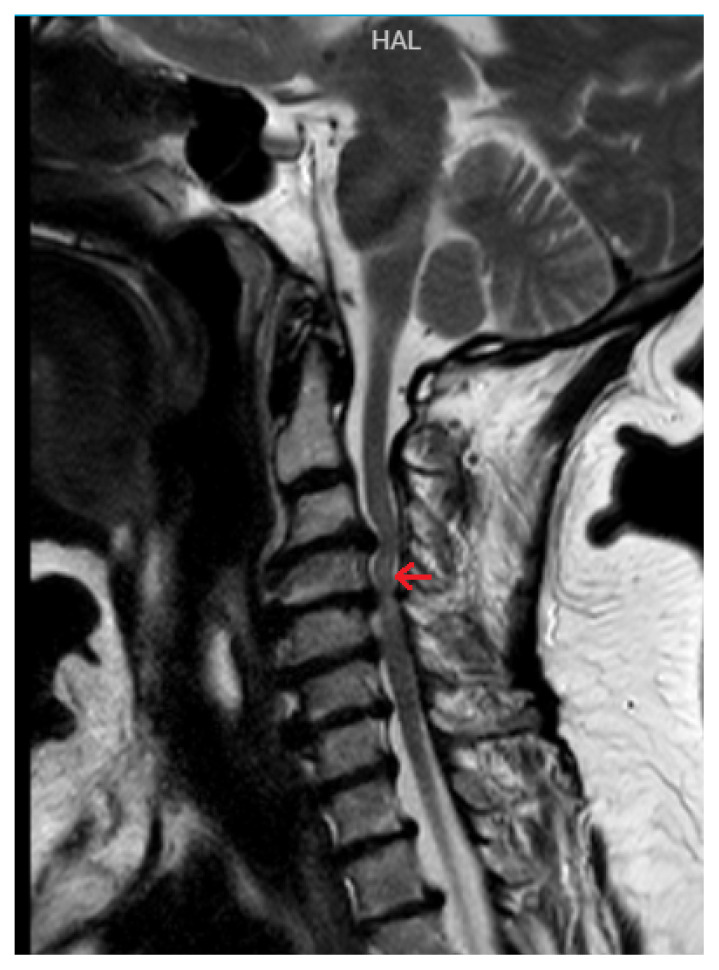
Magnetic resonance imaging of the patient with cervical spine myelomalacia due to disc herniation. Red arrow: Cervical spinal cord myelomalacia.
